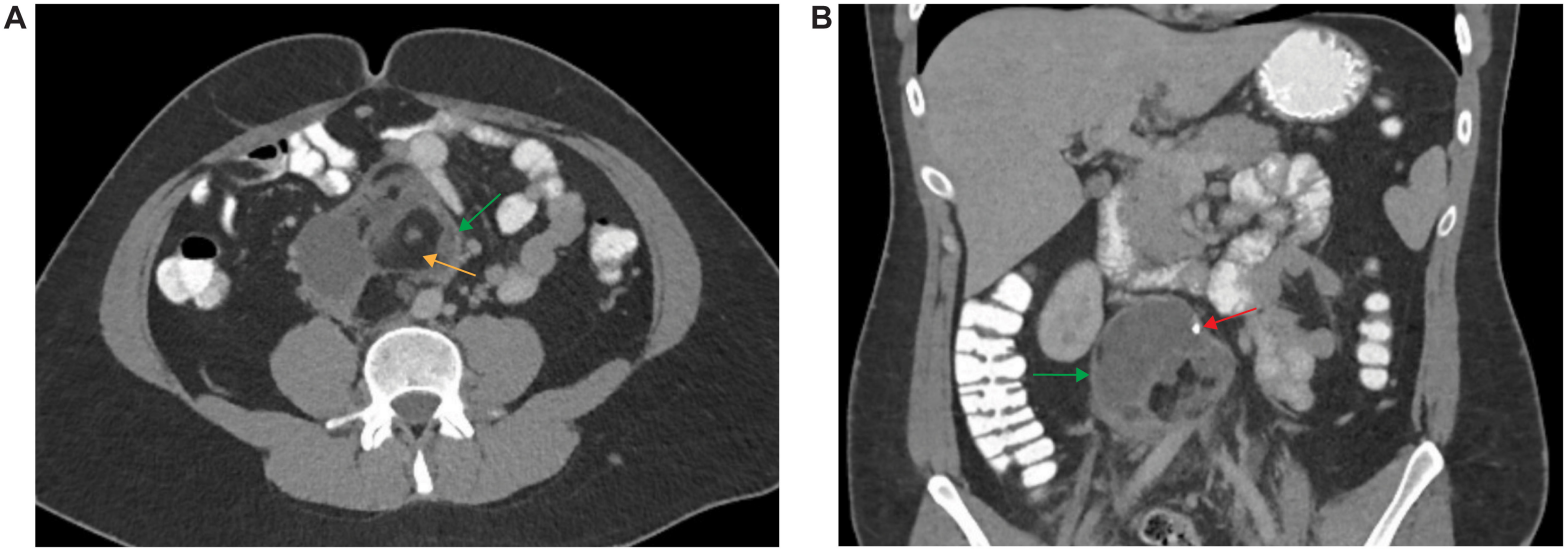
Imaging of Retroperitoneal Mass. CT angiogram of the chest, abdomen, and pelvis with axial (A) and coronal (B) sections depicting a retroperitoneal mass (yellow arrows) containing macroscopic fat (orange arrow) and coarse calcifications (red arrow).
